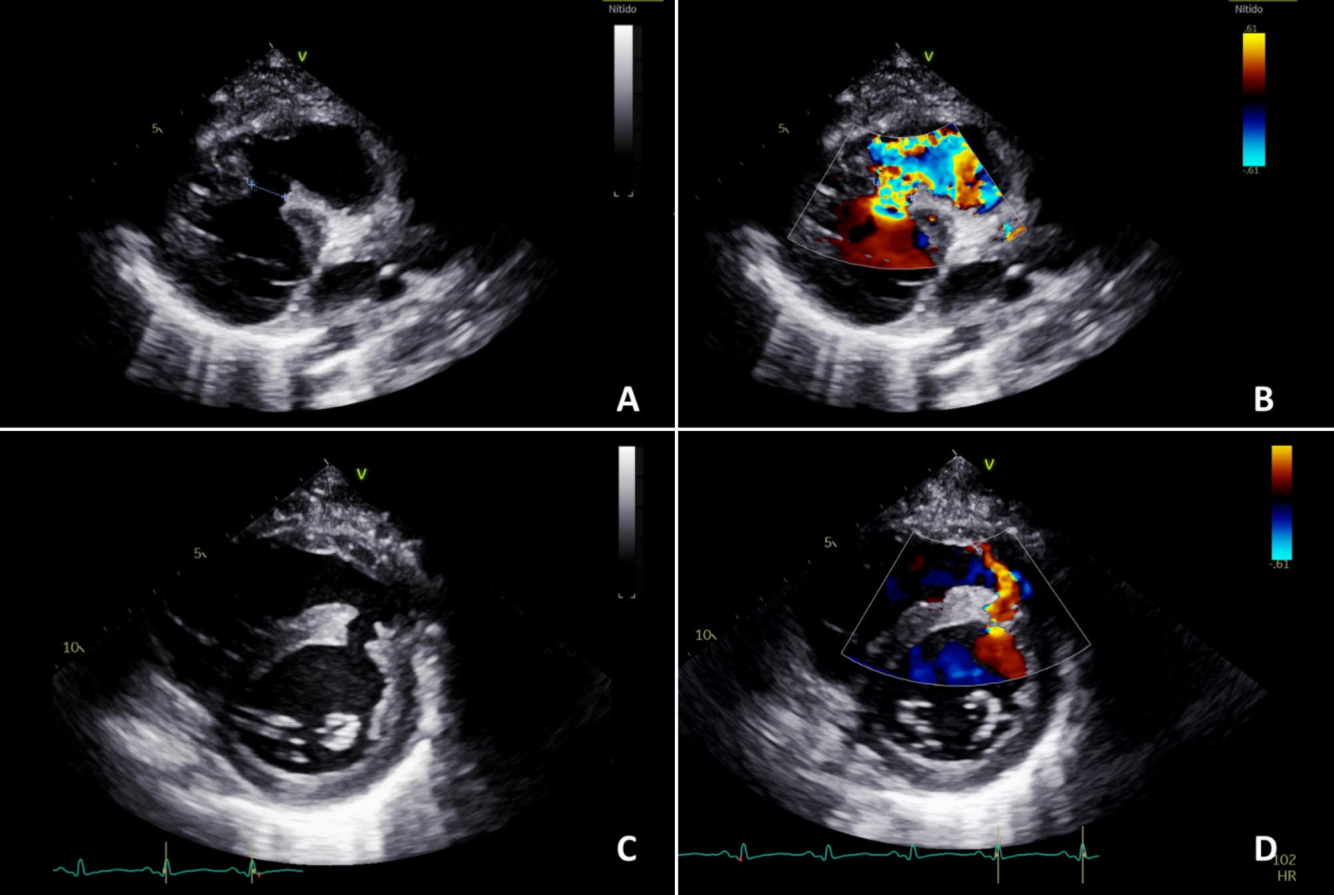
Transthoracic echocardiogram. A. Parasternal long-axis view: 13 mm ventricular septal defect (VSD). B. Color Doppler: Left-to-right shunt across the VSD C. Parasternal short-axis window: VSD D. Color Doppler showing flow through the VSD
